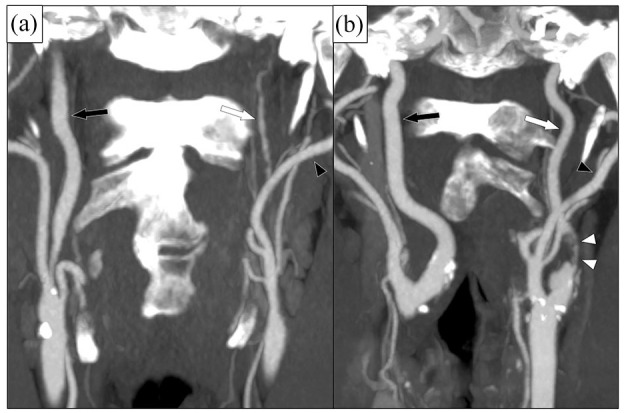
Two cases of left-sided CNO, coronal view on CTA. (a) CNO with full collapse. After a severe stenosis (not in plane), the distal ICA (white arrow) is very small (threadlike), clearly smaller than contralateral ICA (black arrow) and ipsilateral ECA (black arrowhead). (b) CNO without full collapse. After a severe and quite long stenosis (white arrowheads), the distal ICA (white arrow) is normal-appearing, but smaller than contralateral ICA (black arrow) and similar as ipsilateral ECA (black arrowhead).
